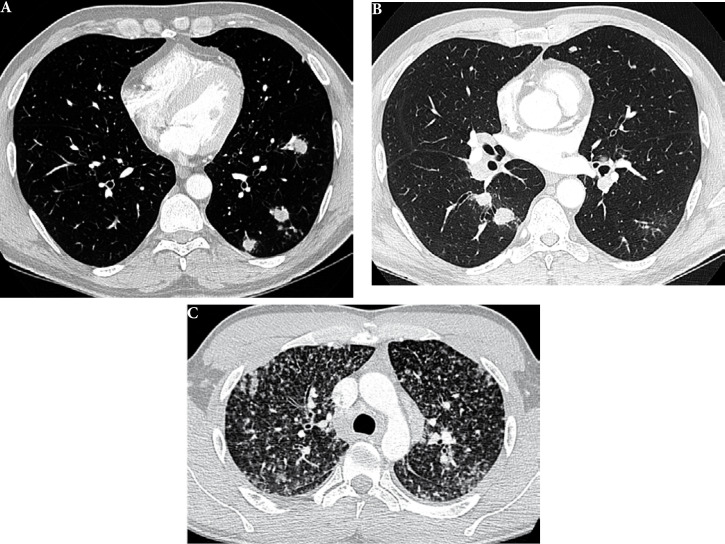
Scanners thoraciques d'histoplasmoses pulmonaires (crédit photo: M. Zappa) Légende: A et B. Scanner thoracique en fenêtre parenchymateuse d'une histoplasmose pulmonaire chez un militaire immunocompétent. Nodules multifocaux bilatéraux du parenchyme pulmonaire. C. Histoplasmose pulmonaire diffuse compliquée de SDRA chez un jeune Guyano-brésilien ayant inhalé une quantité massive de poussière de guano de chauves-souris CTs of pulmonary histoplasmosis (photo credit: M. Zappa) A and B. Parenchymal window chest CT of pulmonary histoplasmosis in an immunocompetent military man. Bilateral multifocal nodules of the lung parenchyma. C. Diffuse pulmonary histoplasmosis complicated by ARDS in a young Guyano-Brazilian man who inhaled massive amounts of bat guano dust
